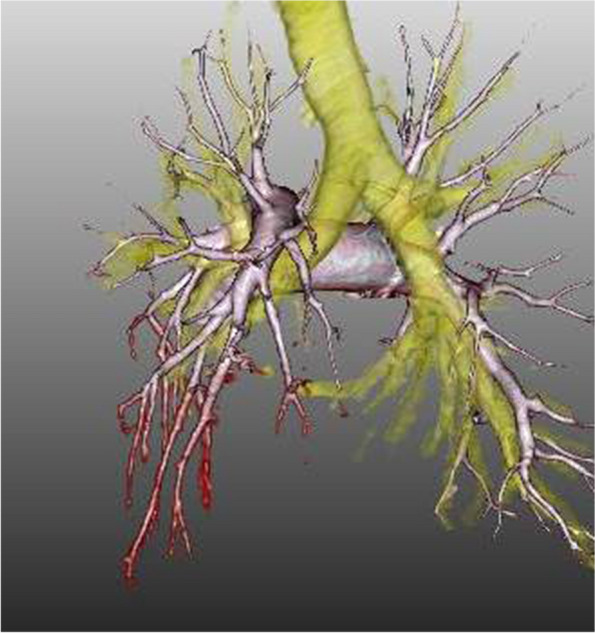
3D CT could identify no findings that cause bleeding, such as lung cancer, pulmonary aneurysm, and arteriovenous malformation
